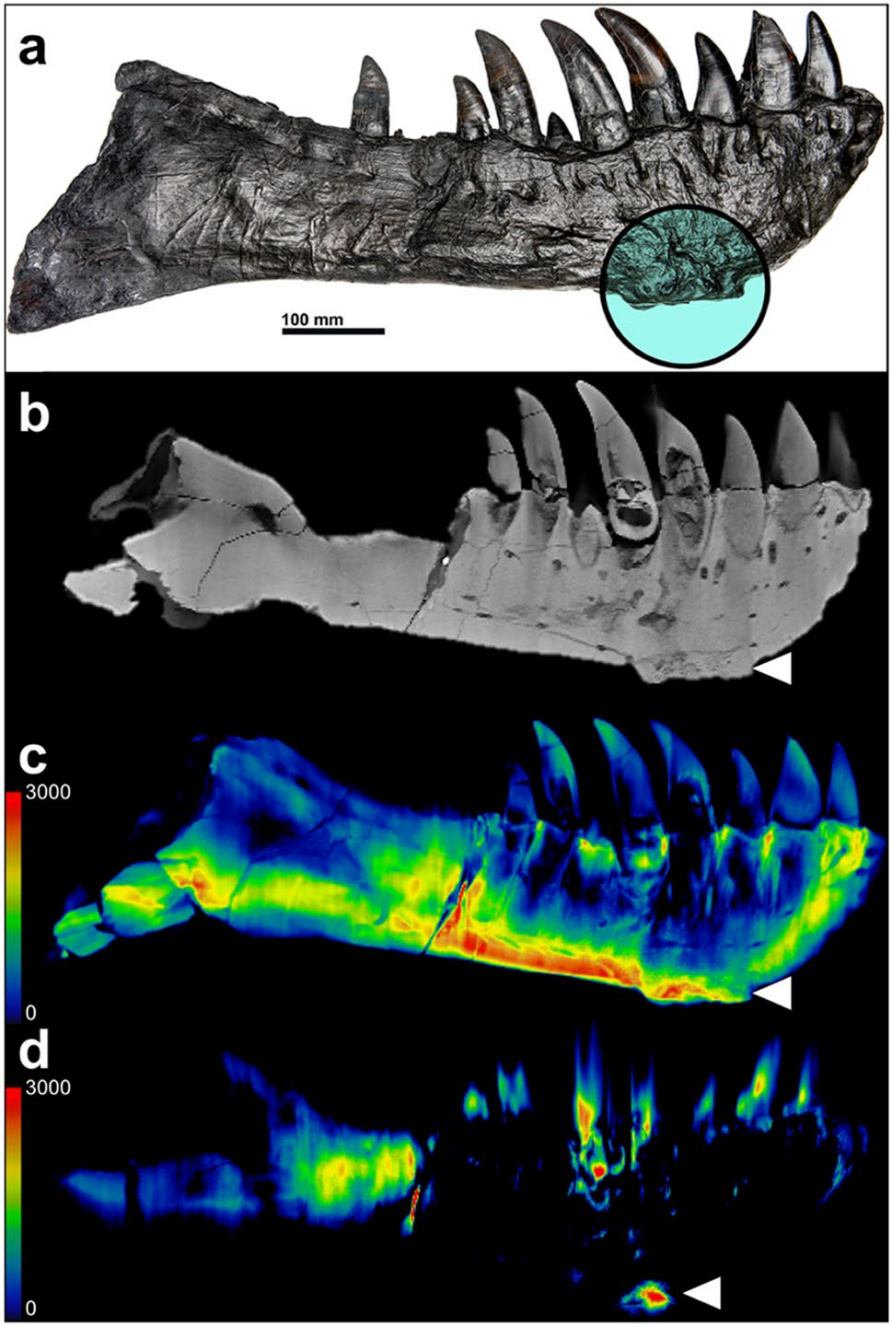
Diseased left dentary of the *Tyrannosaurus rex* MB.R. 91216. Photograph (a), conventional CT-reconstruction (b) and DECT-based calcium (c) and fluorine maps (d) of the left dentary in sagittal orientation. The circle in (a) and the arrowheads in (b–d) indicate the focal exophytic mass on the ventral bone surface at the level of the 3rd to 5th tooth-root. The DECT-material maps demonstrate a diffuse and heterogenous calcium concentration (c) but a focal fluorine accumulation in the central part of the exophytic mass (d). Red indicates high concentrations of the investigated element measured in Hounsfield units (color bar in the left lower corner).
